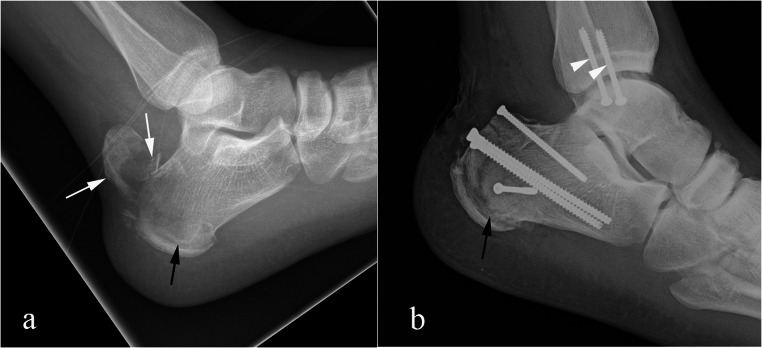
Type I calcaneal tuberosity avulsion fracture. 26-year-old man with a comminuted calcaneal tuberosity avulsion fracture (arrows) after a fall from height (a). Follow up (b) after fixation with multiple screws. The fracture extended inferiorly involving the entirety of the calcaneal tuberosity (black arrows in a and b). CT was not obtained but likely would have resulted in improved visualization of the inferior extent of the fracture (black arrows in a and b). Note is made of screw fixation (arrowheads) of a medial malleolus fracture in b
